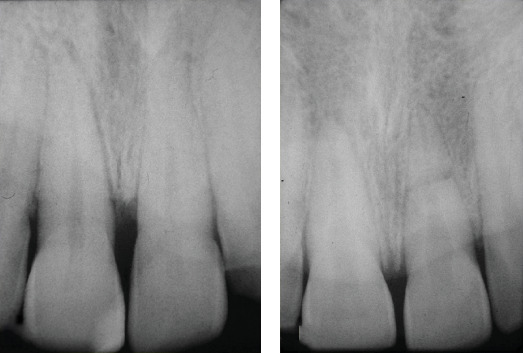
(a) In the initial periapical radiograph, no signs of fracture were observed. (b) However, after changing the orientation of the x-ray beam, the presence of a horizontal fracture became evident.
